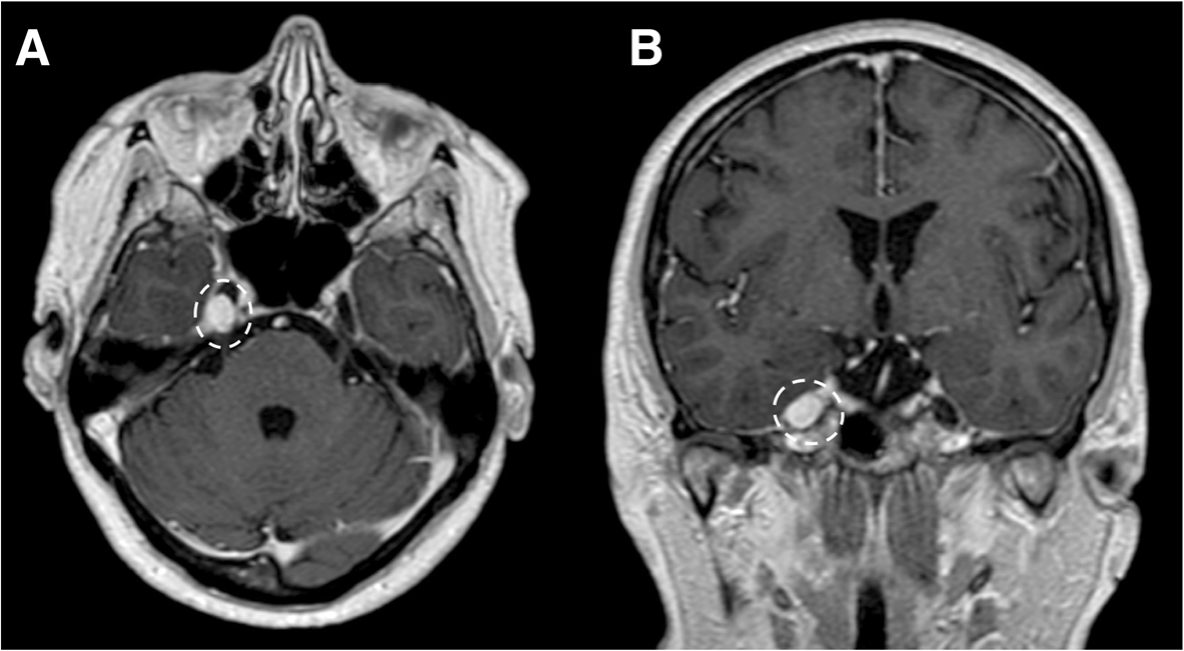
Small trigeminal schwannoma. MRI T1-weighted post-gadolinium axial (a) and coronal (b) images show a homogeneous enhancing mass in the right Meckel’s cave (dotted circles)
